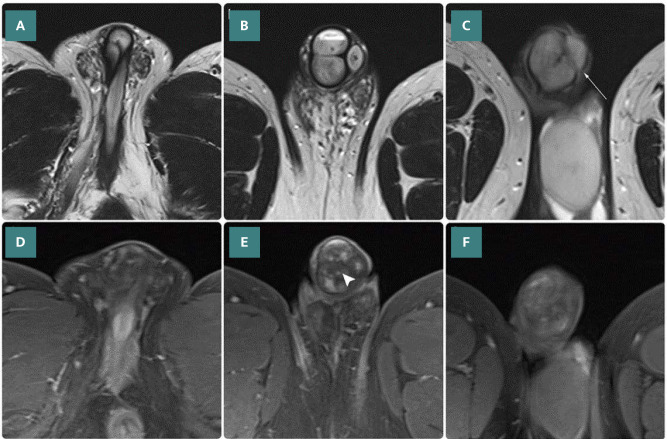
Axial MRI scan of the penis obtained without and with contrast. A, B, and C. T2-weighted sequences obtained at the root (A), body (B), and head (C) levels clearly show counterclockwise torsional twisting of the three erectile structures (corpora), starting from the root (A) and continuing in a 180˚ right-sided torsion. The corpora cavernosa (reversed right to left) and the corpus spongiosum (uplifted) are seen (arrow). D, E, and F. T1-weighted post-contrast image with fat saturation sequence shows heterogeneous enhancement of the corpora and glans penis, corresponding to areas of decreased perfusion and tissue ischemia (arrowheads).
